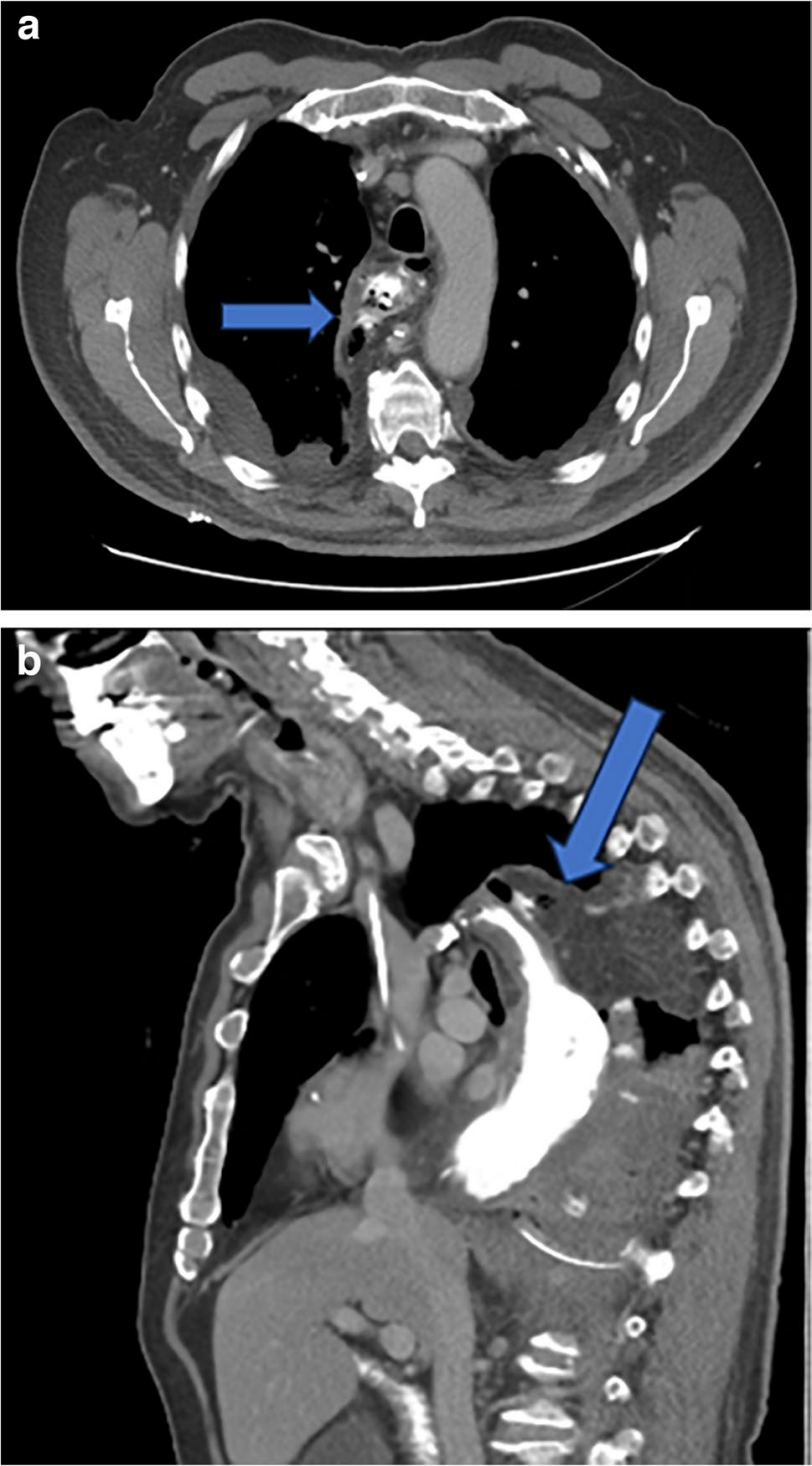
Computed tomography after esophagectomy showing anastomotic leakage. A 77-year-old man operated with minimally invasive esophagectomy using the Ivor Lewis technique. Postoperative day 5, anastomotic leakage (arrows) was depicted in computed tomography with oral contrast, axial (a), and sagittal (b) reformations
